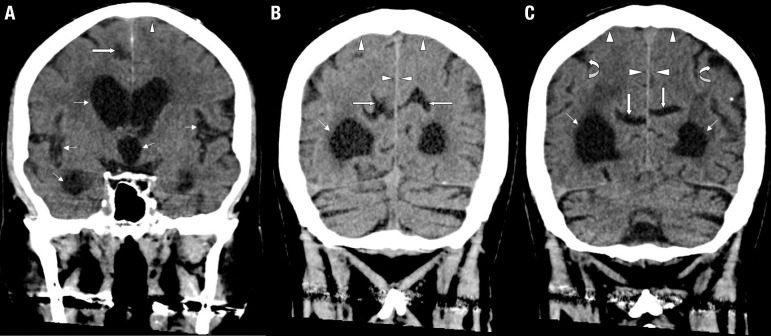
Coronal head CT of a 73-year-old man with idiopathic NPH. [A, B and C]
